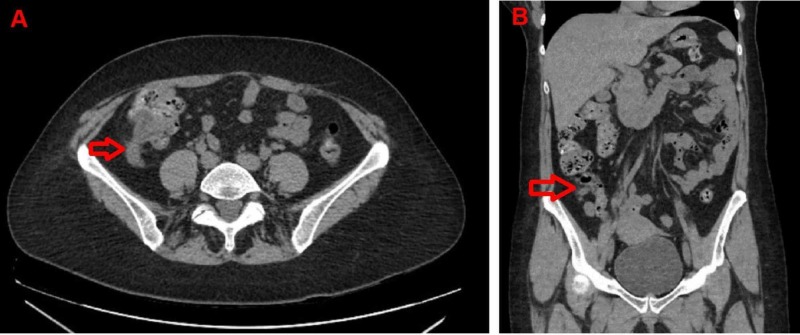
Computed tomography (CT) of the abdomen A – Axial CT scan image, and B – Coronal CT scan image. Arrows show dilated appendix measuring up to 1.1 cm with mild periappendiceal inflammatory fat stranding.
